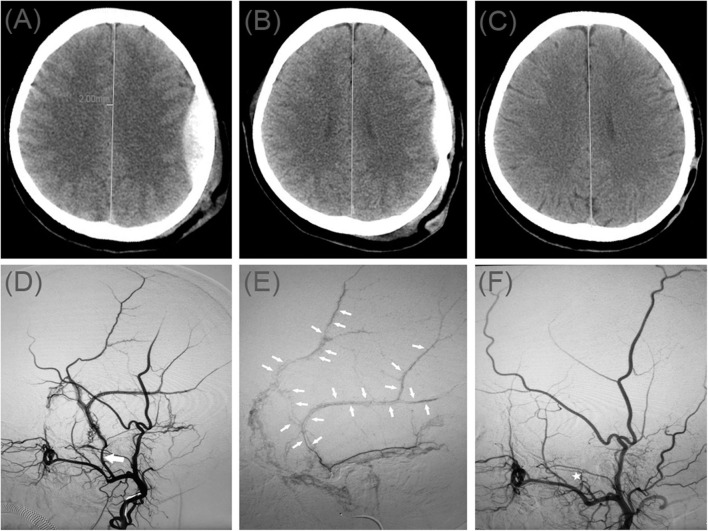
(A) Brain computed tomographic hematoma images for patient 1 before treatment (midline shift: 2.00 mm). (B) Brain CT was repeated on postoperative day 11. (C) Follow-up brain CT was performed at 88 days. (D) Pre-operative DSA presented abnormal MMA (Large arrow). (E) Intra-operative DSA showed contrast agent extravasation or arteriovenous fistula (Small arrow). (F) Postoperative DSA found that the MMA disappeared (Asterisk). DSA, digital subtraction angiographic.
